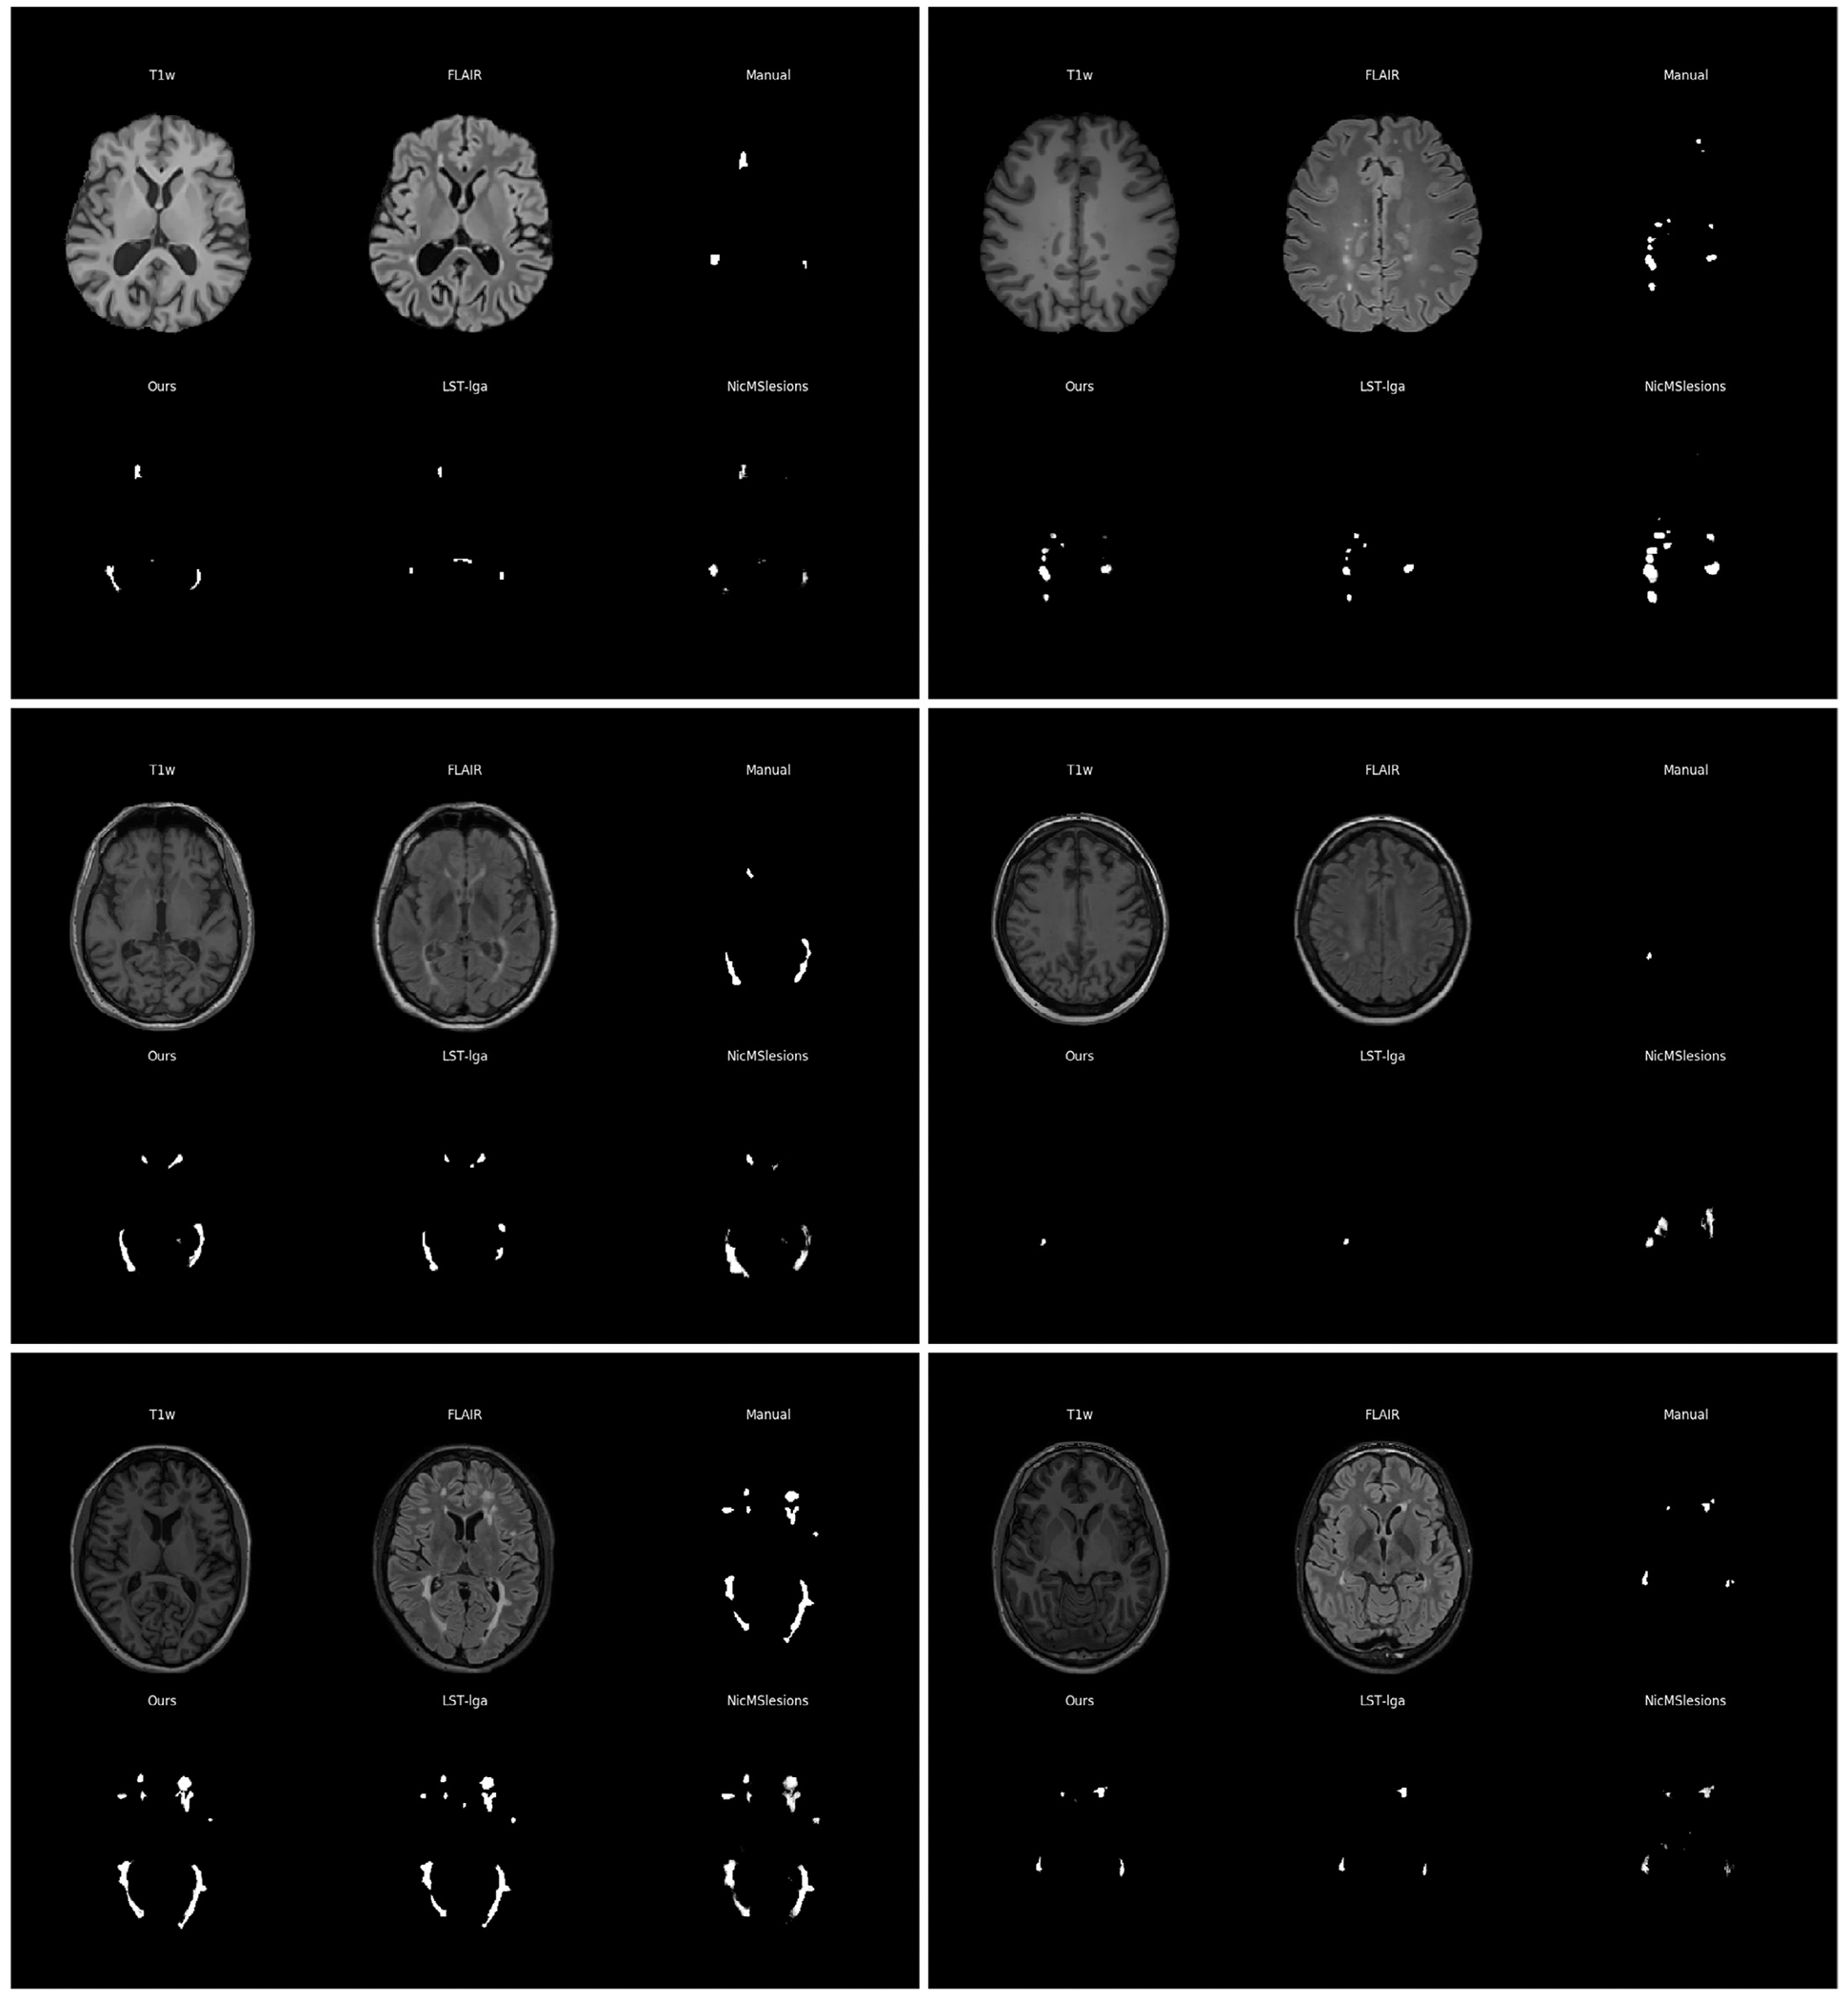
Visual comparison of lesion probability maps on three different datasets for the proposed method and two state-of-the-art lesion segmentation methods (LST-lga and NicMsLesions) on T1w-FLAIR input. (Top) Two subjects from the MSSeg dataset; (Middle) Two subjects from the Trio dataset; (Bottom) Two subjects from the Achieva dataset. For each subject the top row shows slices of the data and the manual annotation while the bottom row shows the lesion probability maps for our model, LST-lga and NicMsLesions.
